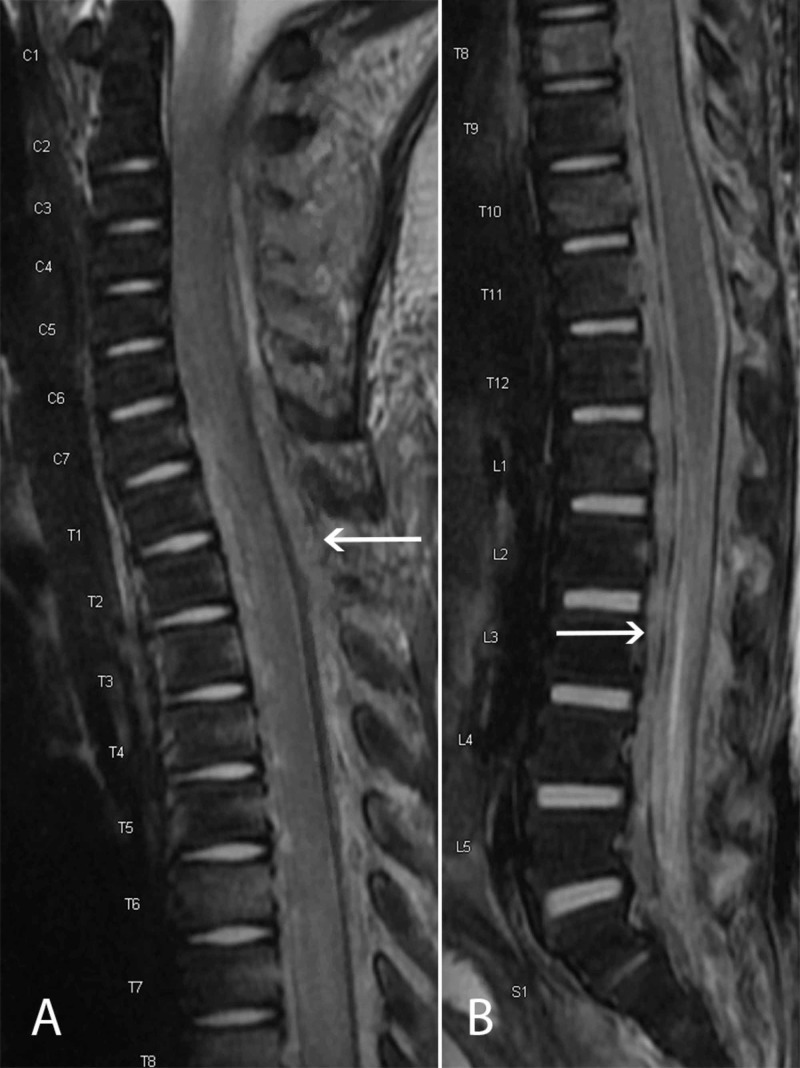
Interval T2 MRI following initial decompression with recurrent/residual extensive spinal epidural abscess in the cervicothoracic (A) and lumbosacral (B) regions
